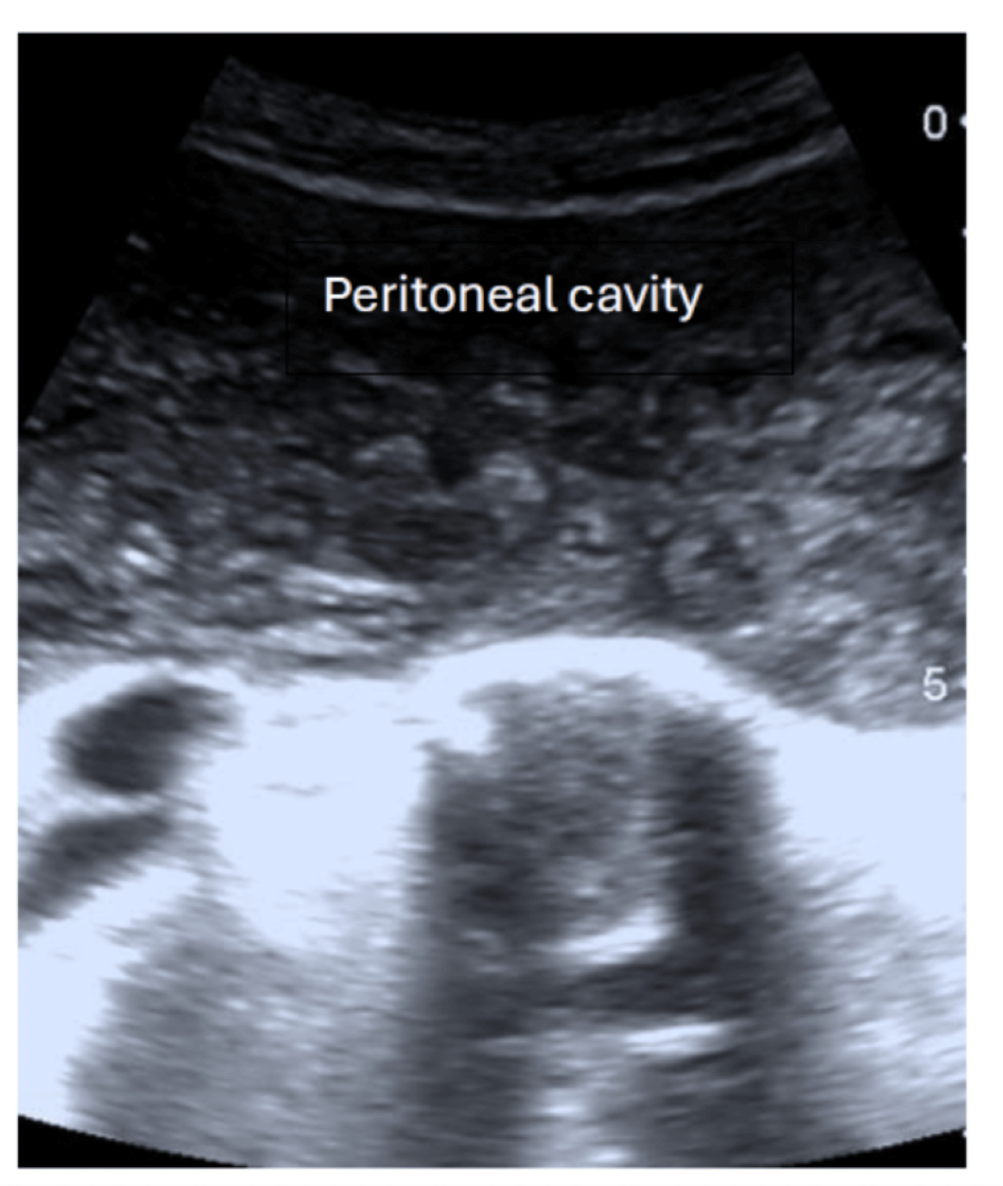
Ultrasound of the abdomen showing peritoneal cavity with no cysts after treatment.
